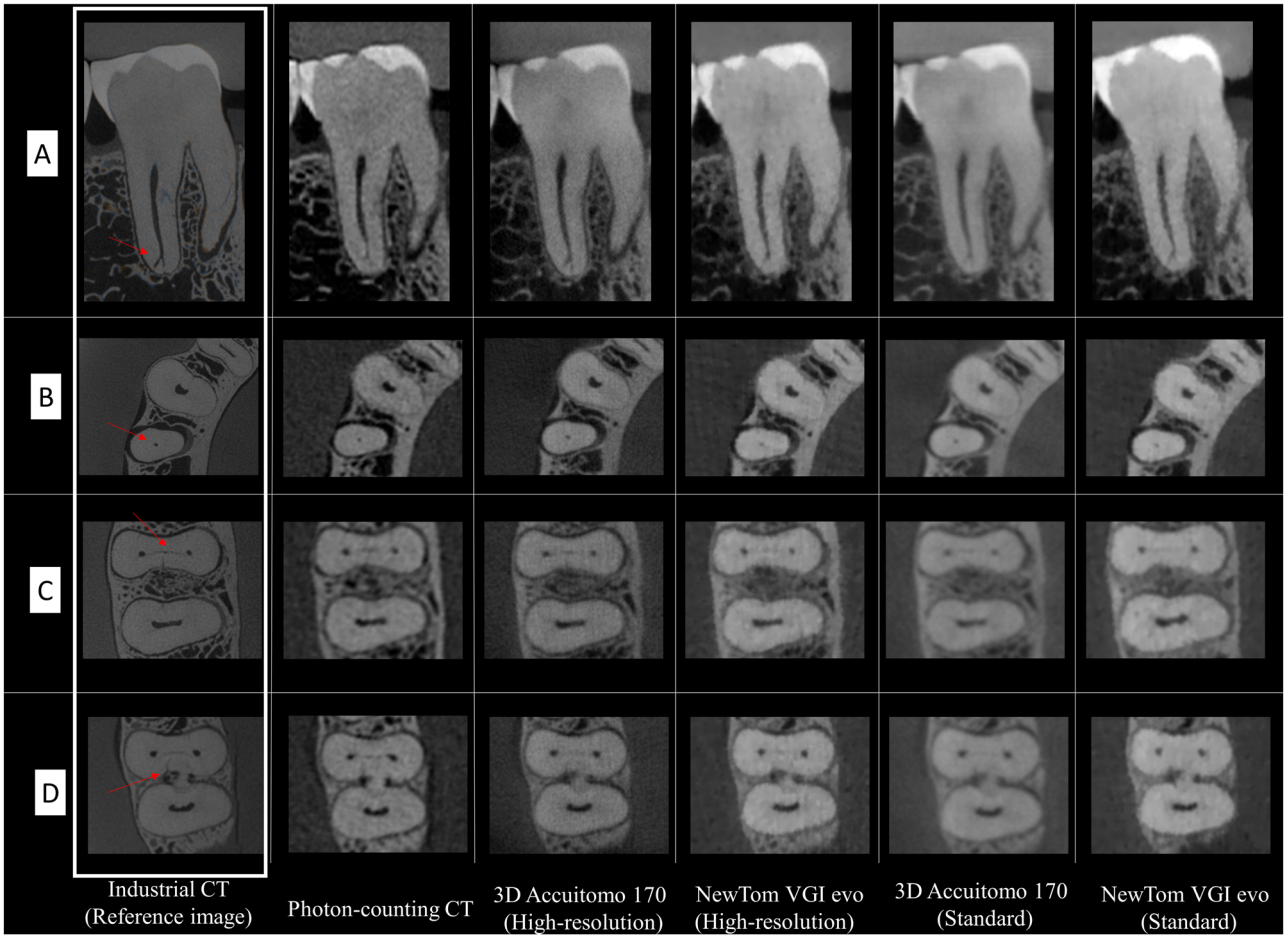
Representative imaging reconstructions of each diagnostic condition (as indicated by the arrows in the set of images of the Industrial CT—reference image) according to the different imaging modalities tested: (A) Apical delta; (B) Narrow canal; (C) Isthmus; (D) Root crack.
